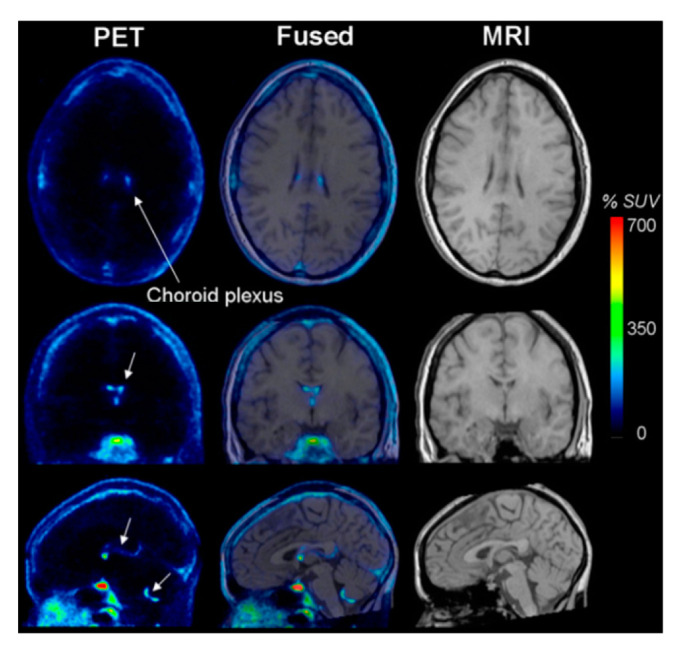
PET Image. Brain image taken using positron emission tomography (PET) and T1 MRI images. Red regions of the image show areas of tracer accumulation. The fused image is the PET image superimposed on the structural MRI image. http:/doi:10.2967/jnumed.108.058453, accessed on 24 April 2022.
